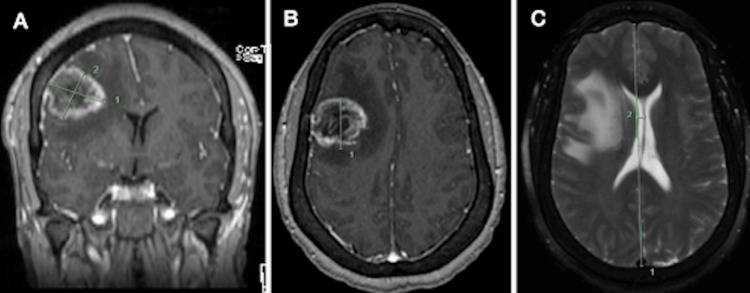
MRI brain demonstrating a 3.5 x 3.0 x 3.3 cm mass centered within the right frontal opercular region with a large amount of surrounding vasogenic edema, leading to approximately 5 mm of leftward midline shift with slight effacement of the right lateral ventricle. (A) Coronal view demonstrating the mass in the right frontal opercular region. (B) Axial view demonstrating the mass with surrounding vasogenic edema. (C) Axial view showing 5 mm of leftward midline shift.
